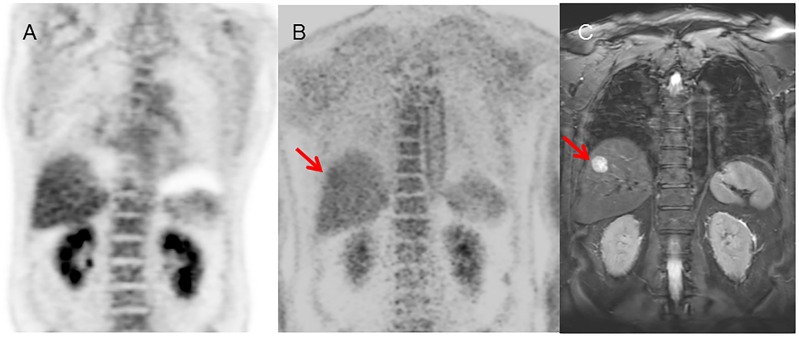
Lesion identified prospectively on PET/MRI but only retrospectively on PET/CT. 66 year-old male with neuroendocrine tumor who underwent PET/CT for initial staging (same patient as in Fig 3). (A) PET image from PET/CT, (B) PET image from PET/MRI, (C) MRI (STIR) image. This liver metastasis could be clearly identified on PET/MRI because of the T2-bright lesion seen on MR STIR imaging. The lesion has only mildly increased activity compared to the normal liver, and was not evident prospectively due to the poor soft tissue contrast of the CT scan from the PET/CT study.
